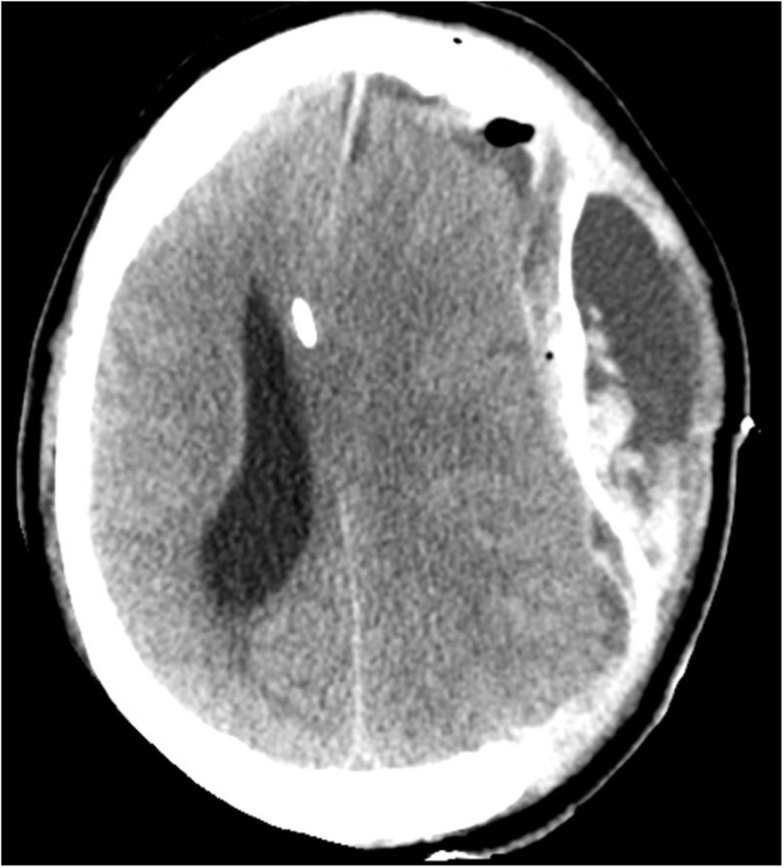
CT on day 11, 4 days post a left-sided hemicraniectomy. An EVD catheter *in situ* in the right hemisphere. There is a left-sided epidural expansive mass adjacent to the duraplasty (artificial membrane) causing a severe midline-shift.
